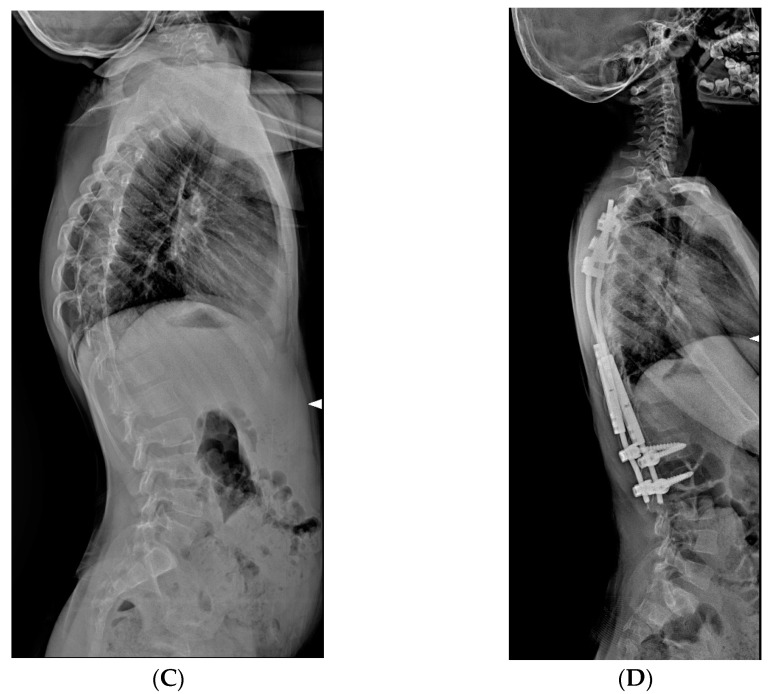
X-rays of early onset scoliosis (EOS) corrected with traditional growing rods (TGR): (A) pre-operative antero-posterior X-ray view; (B) post-operative antero-posterior X-ray view; (C) pre-operative lateral X-ray view; (D) post-operative lateral X-ray view.
